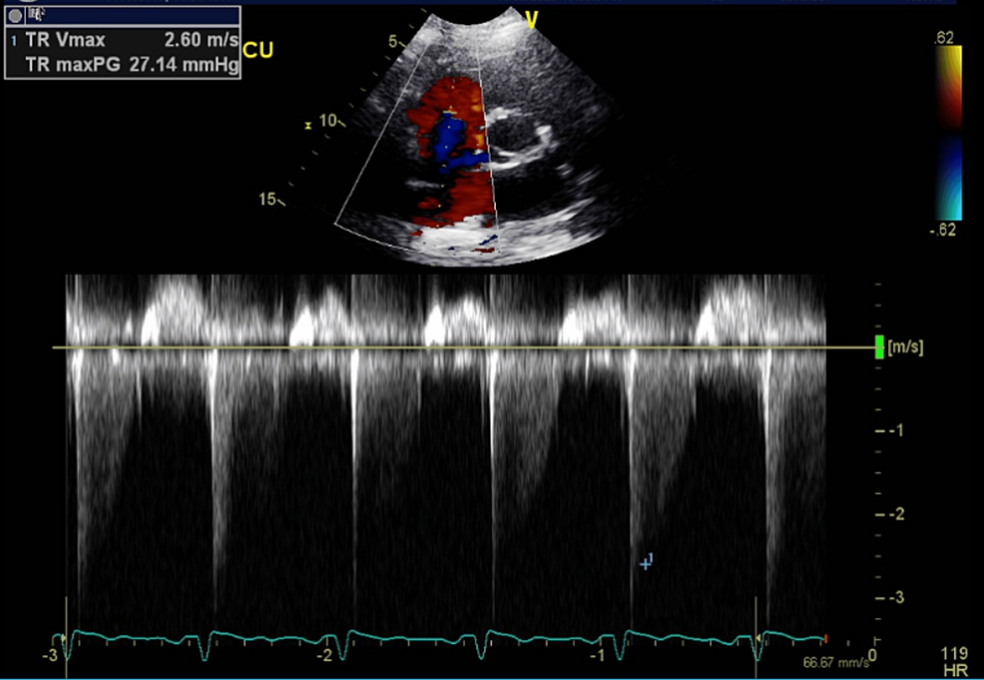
Follow-up transthoracic echocardiography after stabilization - image 3 Transthoracic echocardiography; apical 4-chamber view shows improvement in the degree of tricuspid regurge (TR Vmax: 2.60 m/s, TR maxPG: 27.14 mmHg)
